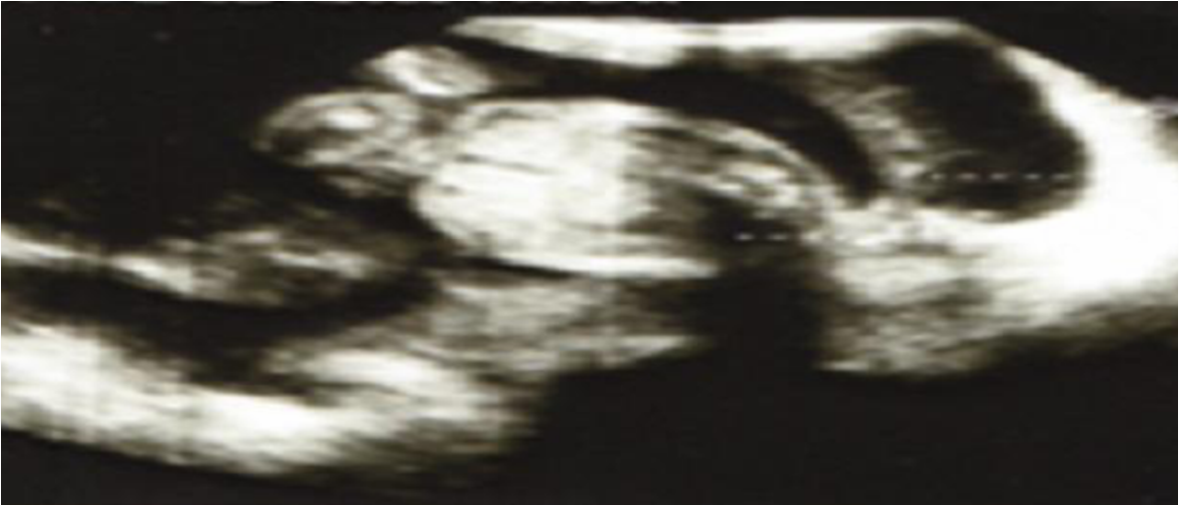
Obstetric ultrasound image showing a pregnancy at 22 weeks of amenorrhea with ascites.
